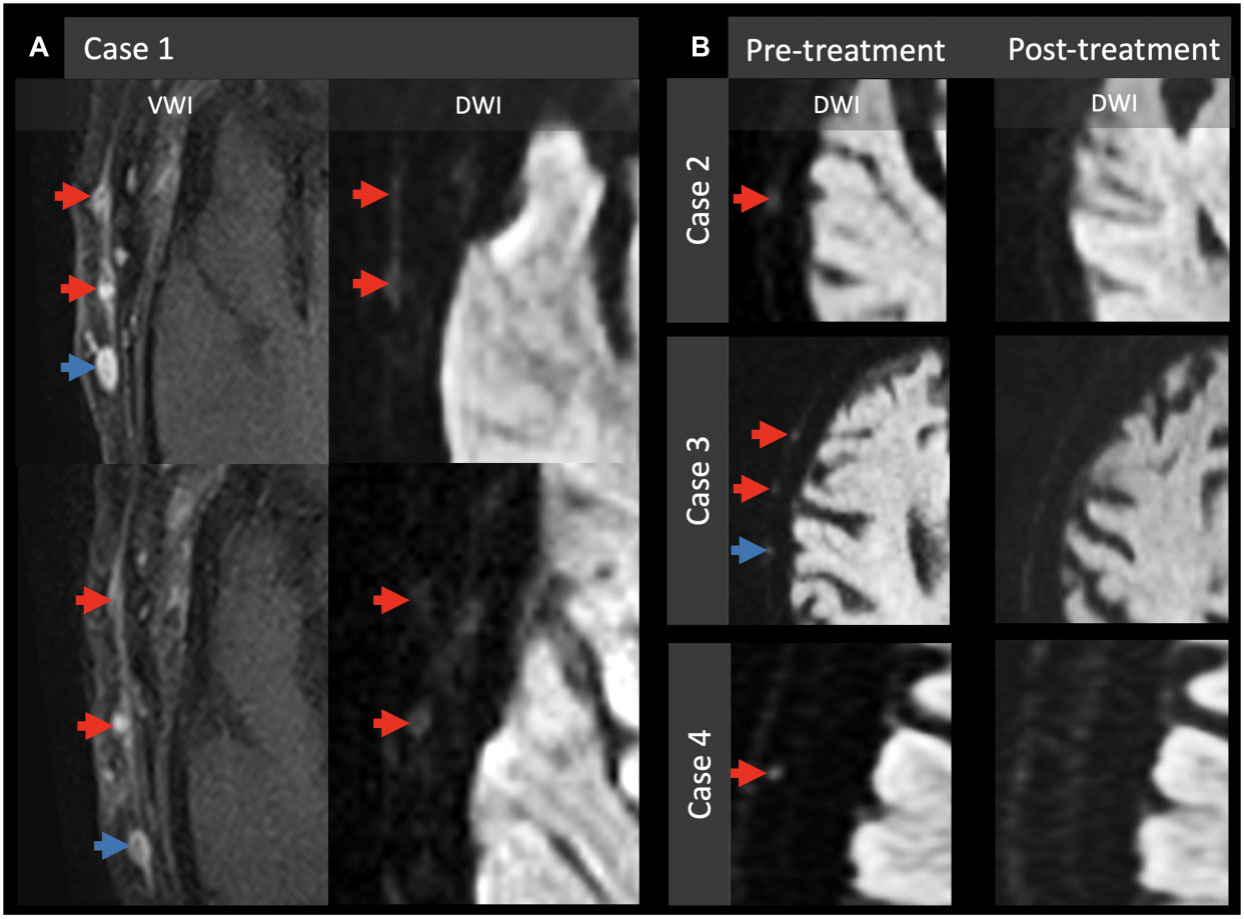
Four patients with GCA all demonstrating restricted diffusion related to the superficial temporal arteries. (A) Left, MRI of case 1 at presentation showing concentric wall thickening and enhancement of the right STA branches on VWI. Right, DWI of the same regions demonstrating high DWI signal and low apparent diffusion coefficient (not shown) in the same STA branches. (B) Three further cases where restricted diffusion is demonstrated in the STA branches (left column), which resolved on the post-therapy MRI (right column). Red arrows, STAs; blue arrows, superficial temporal veins. DWI: diffusion-weighted imaging; STA: superficial temporal artery; VWI: vessel wall imaging
